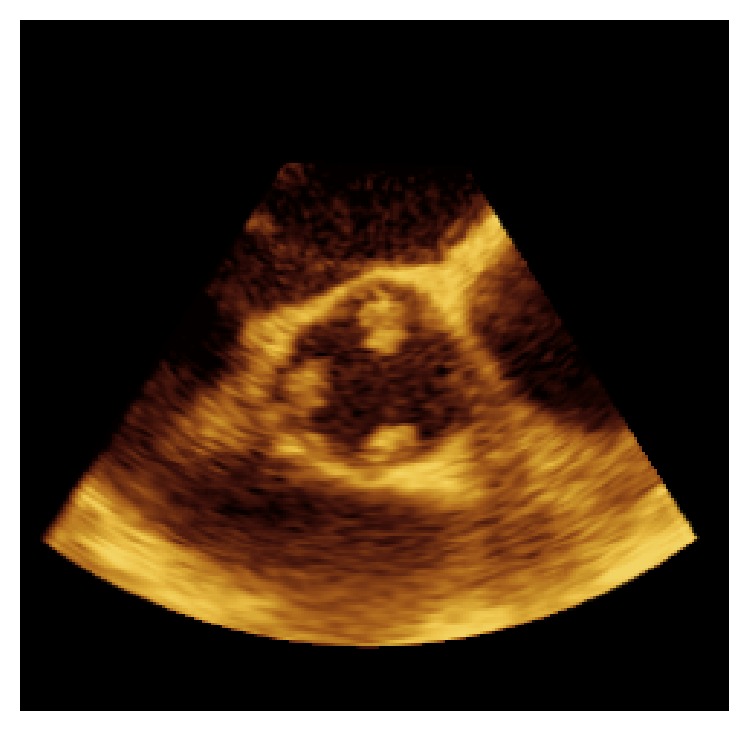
2D aortic valve short-axis, transesophageal view displaying fungal vegetation on all three aortic valve leaflets.
